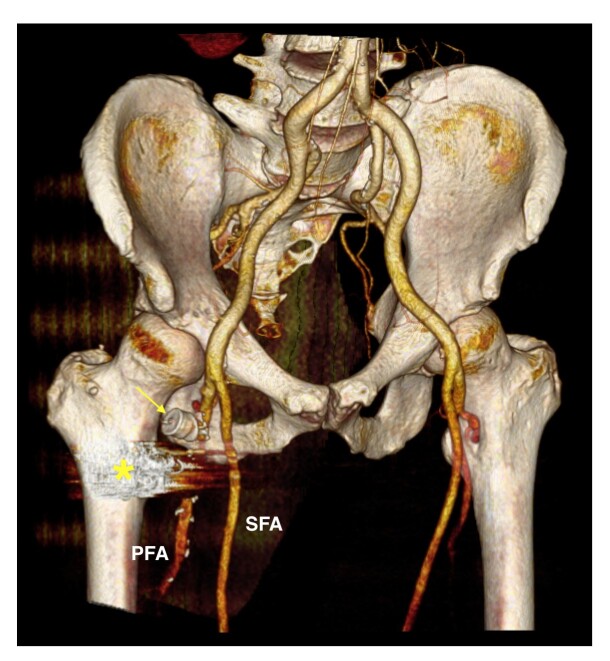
CTA - 3D Volume Rendering: The control CT scan shows the patent profunda femoris artery, the vascular plug at the origin of the circumflex artery (arrow), and the artifact caused by the coils (*) within the aneurysmal sac, which is completely thrombosed. CTA: Computed tomography angiography; PFA: Profunda femoris artery; SFA: Superficial femoral artery.
